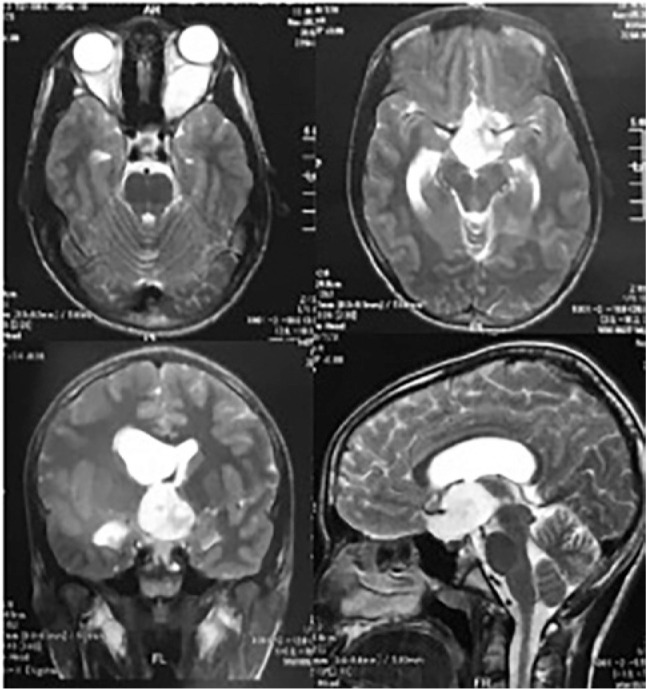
T1-weighted Gd-enhanced magnetic resonance images demonstrating a
